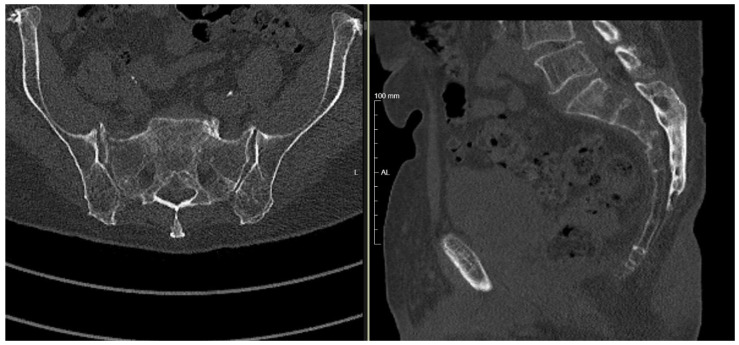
Preoperative CT imaging of an H-type sacral insufficiency fracture (FFP IVb). Axial (left) and sagittal (right) reconstructions demonstrate the fracture configuration of the posterior pelvic ring.
